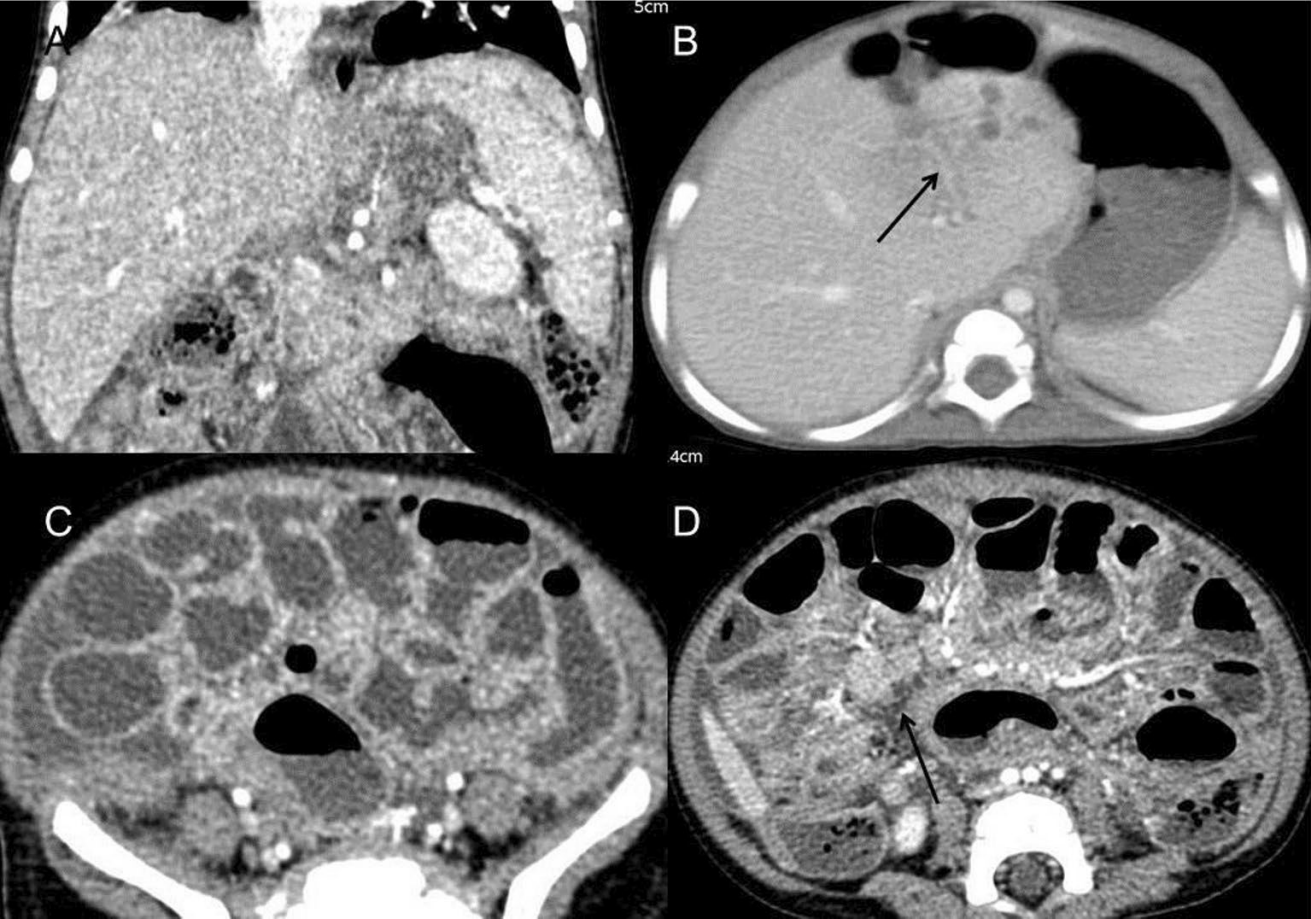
Abdominal visceral involvements of BCG infection. (A) Coronal post-contrast CT showed hepatosplenomegaly with homogeneous enhancement in a 1-year-old boy. (B) A 8-month-old boy with hepatic abscess. CT scan demonstrated the presence of multiple low-attenuated lesions in the left lobe (long arrow). Most lesions were less than 5 mm and some with ring enhancement. Edema around the left portal vein were also noticed. (C,D) A 1-year-old boy with enteritis. The widely thickened intestinal wall and effusion within the lumen were found on abdominal CT. The small amount of ascites also accompanied. The enlargement of mesenteric lymph nodes was noticed (D, long arrow).
